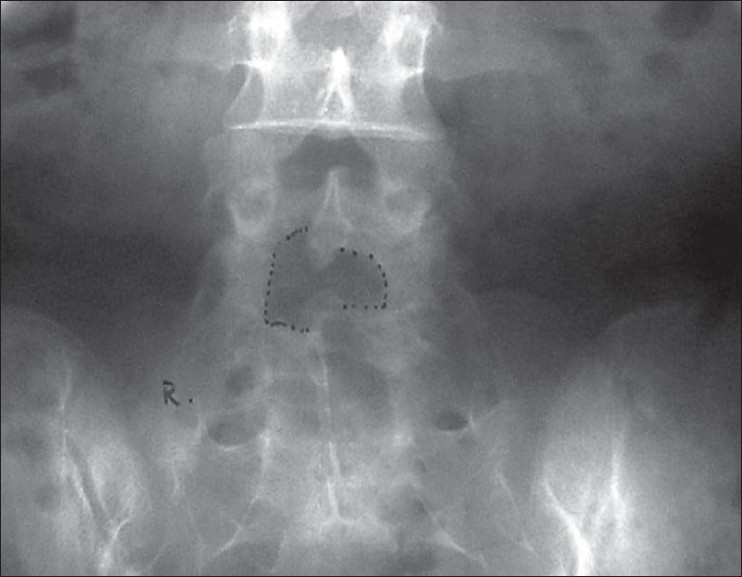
Postoperative X-ray lumbosacral spine anteroposterior view showing size of laminotomy
